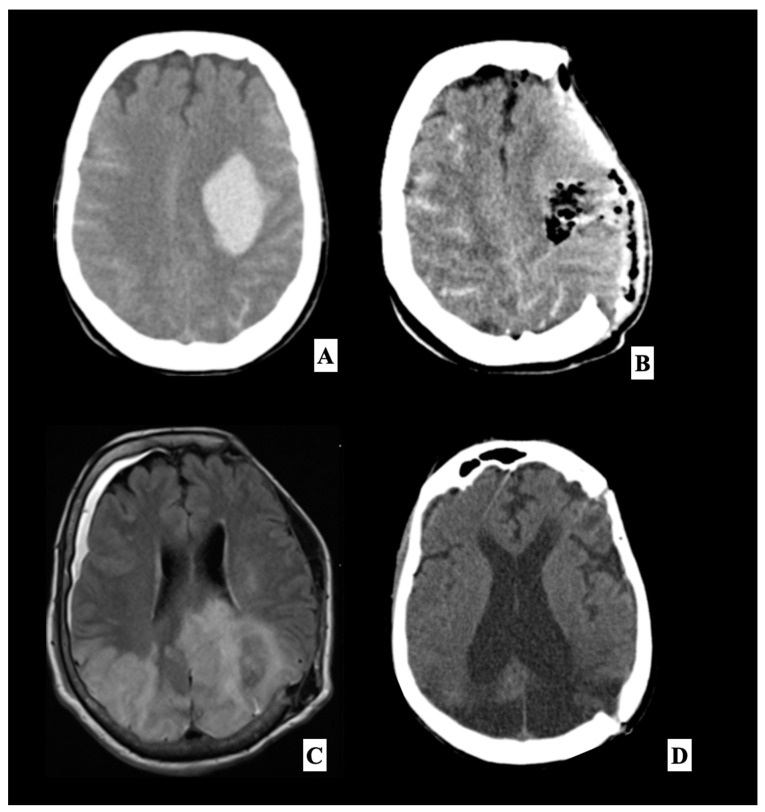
This is a representative case of a 55-year-old woman with no significant previous medical history and essential hypertension, who was transported to our emergency unit after the onset of right hemiplegia with Glasgow Coma Scale (GCS) 6, preceded by a severe headache which occurred two hours earlier. On initial evaluation, blood pressure was elevated at 160/100 mmHg. A head angio computed tomography (A) scan performed at our department showed a large left frontotemporal subdural hemorrhage. A left front-temporoparietal decompressive craniectomy was performed to remove the hematoma. Postoperative head CT scan (B) showed the surgical results, with complete clot removal with a significant reduction in the midline shift. The patient was therefore transferred to our intensive care unit. During sedation, the patient had poorly controlled hypertensive peaks and the causes of secondary hypertension were excluded (urinary catecholamines analysis, abdominal CT-scan and serum-level hormones). The patient was extubated four days after the craniectomy. The neurological examination documented a slight improvement in the right side motor disorder and a considerable improvement in the consciousness state, with a GCS 10. A brain MRI (C) was performed about 3 weeks after surgery, which showed bilateral occipital, parietal and left frontal cortex and subcortical white matter T2/Fluid-attenuated inversion recovery (FLAIR) hyperintensities associated with disruption of the BBB evident after administration of gadolinium, suggestive of PRES. The patient underwent a cranioplasty with her autologous bone flap after three weeks. On follow-up at six weeks, the patient did not present modification of neurological status and reported no recurrence of severe neurological deficits or seizures. She was submitted to an Electroencephalography (EEG) that showed normal, reduced electroencephalographic activity without sign of seizure, and a follow-up head CT scan showed an enlargement of the frontal ventricles (D), and the patient was given third ventriculostomy and a ventriculoperitoneal shunt. After one week, the patient showed a general improvement in verbal and motor response.
